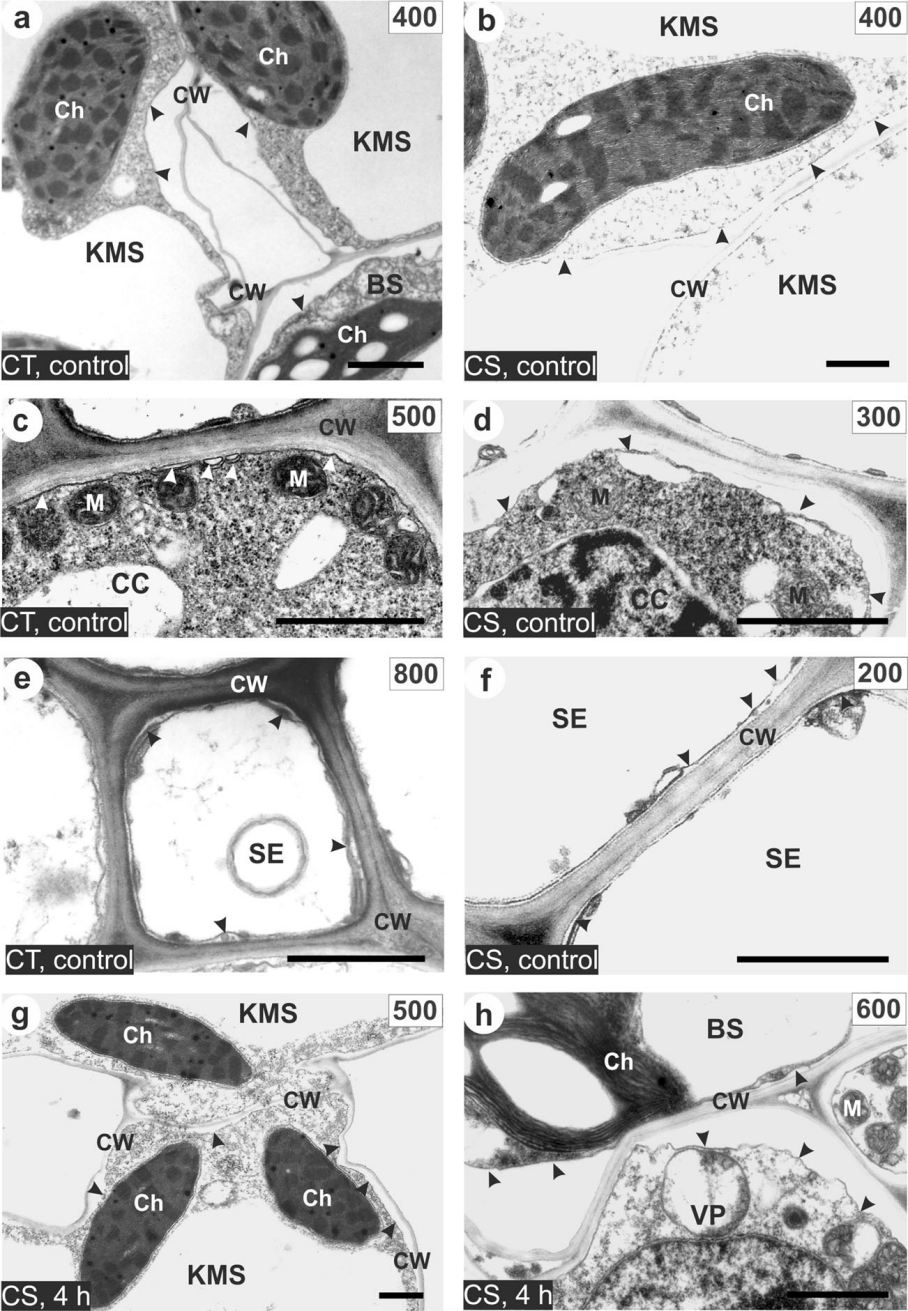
Examples of incipient plasmolysis in cells of control (non-chilled) (a–f) and chilled leaves for 4 h (g, h) of chilling-tolerant (*CT*) and chilling-sensitive (*CS*) maize line incubated in different sorbitol concentration (values in *rectangles*). Note that protoplast is separating from the cell wall (*arrowheads*). *KMS* Kranz mesophyll; *BS* bundle sheath; *VP* vascular parenchyma; *CC* companion cell; *SE* thin-walled sieve tube; *Ch* chloroplast, *M* mitochondria; *CW* cell wall; *Scale bar*, 1 μm
